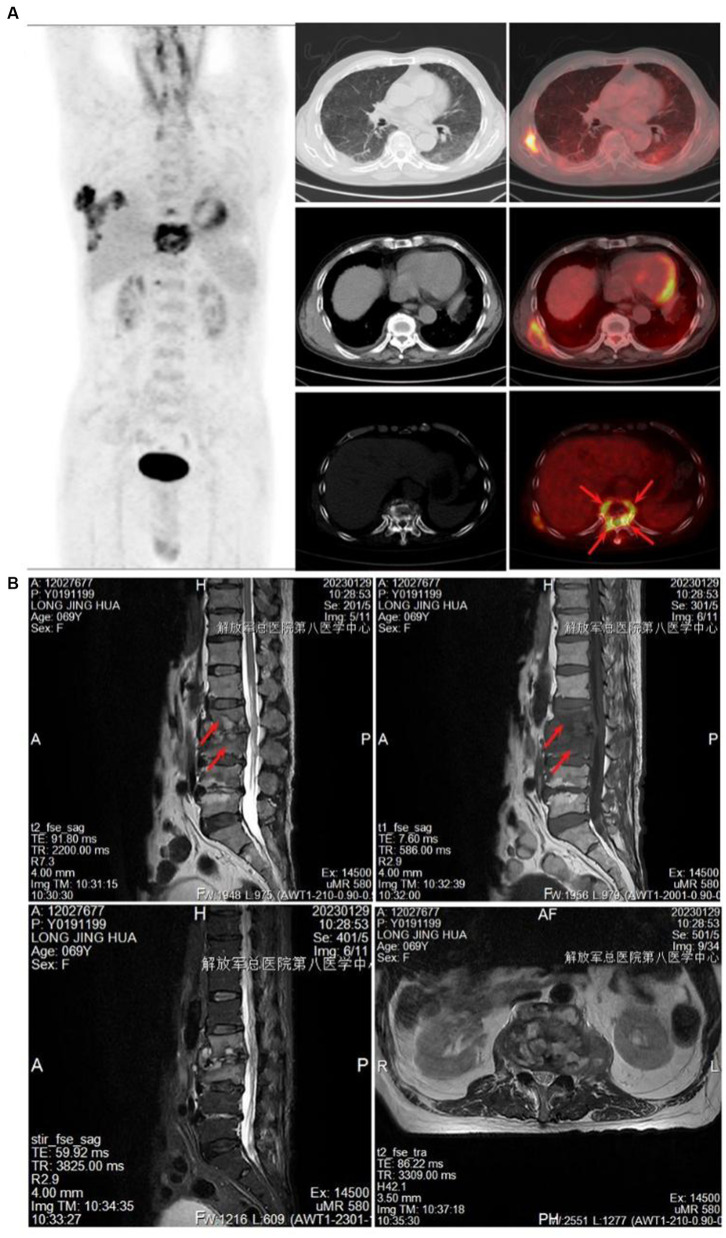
Imaging examination. (A) PET-CT showed increased FDG uptake in the diseased vertebrae. (B) MRI showed infectious lesions in the diseased vertebral body, the formation of paraspinal and bilateral psoas muscle abscesses, and the edema of the surrounding soft tissues.
